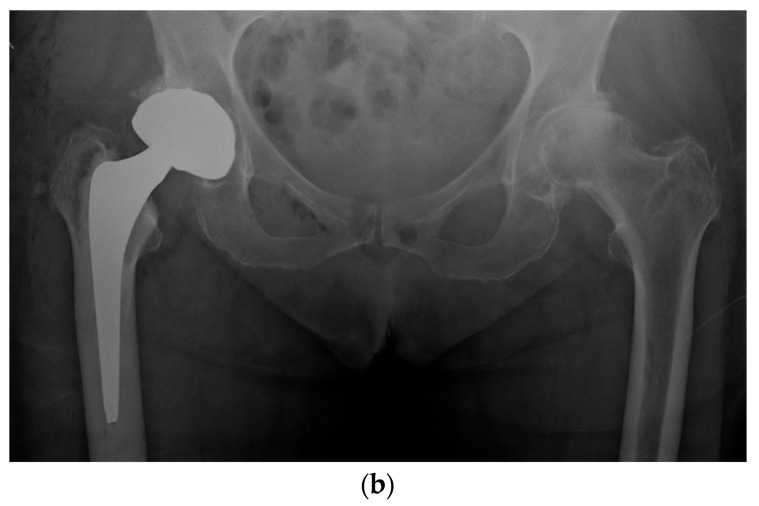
(a) Antero-posterior pelvis radiograph of a 75-year-old woman with severe bilateral hip osteoarthritis. During the right THA surgery, the acetabular cavity was reamed to 47 mm. A monobloc acetabular component of 48 mm was implanted with a DM polyethylene head of 41 mm (28 mm metal head). A polished tapered stem was cemented. (b) Post-operative anteroposterior pelvis radiograph.
